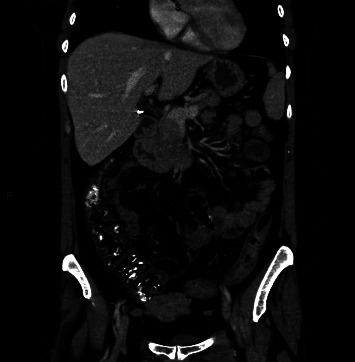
CT showed diffuse colitis.
